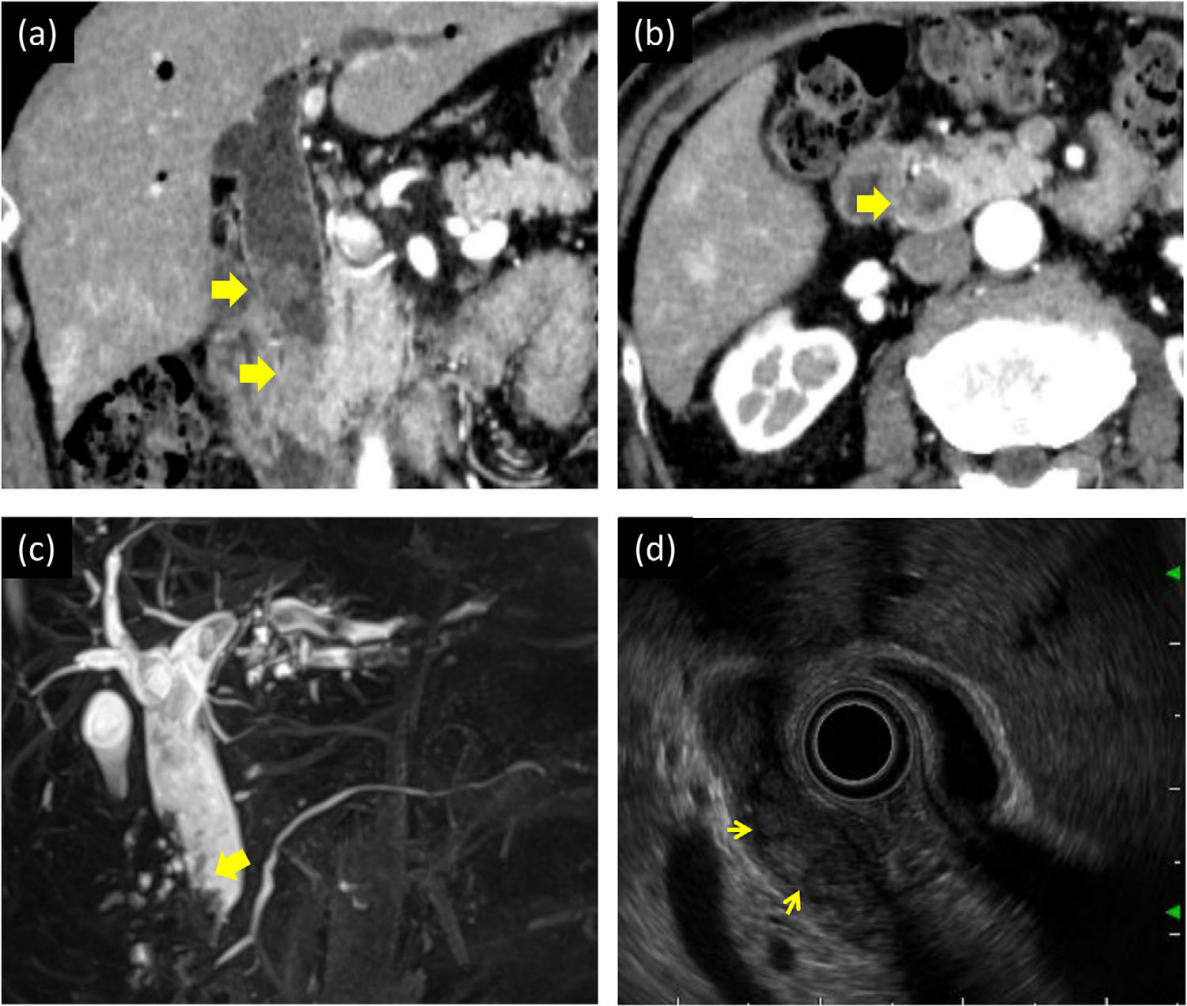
Imaging findings of the mass with low enhancement (yellow arrows), which occupied the middle to lower bile duct, and dilatation of the common bile duct and intrahepatic bile ducts. Coronal (a) and axial (b) abdominal contrast‐enhanced computed tomography images. (c) On magnetic resonance cholangiopancreatography, a tumor‐like defect in the common bile duct (yellow arrow) with dilatation of the intrahepatic and common bile ducts was observed. (d) Endoscopic ultrasonography revealed dilatation of the common bile duct, the elevated papillary tumor in the middle bile duct (yellow arrow), and mucus accumulation in the upper bile duct. The proximal bile duct wall was mildly thickened. The hilar bile ducts could not be fully observed.
